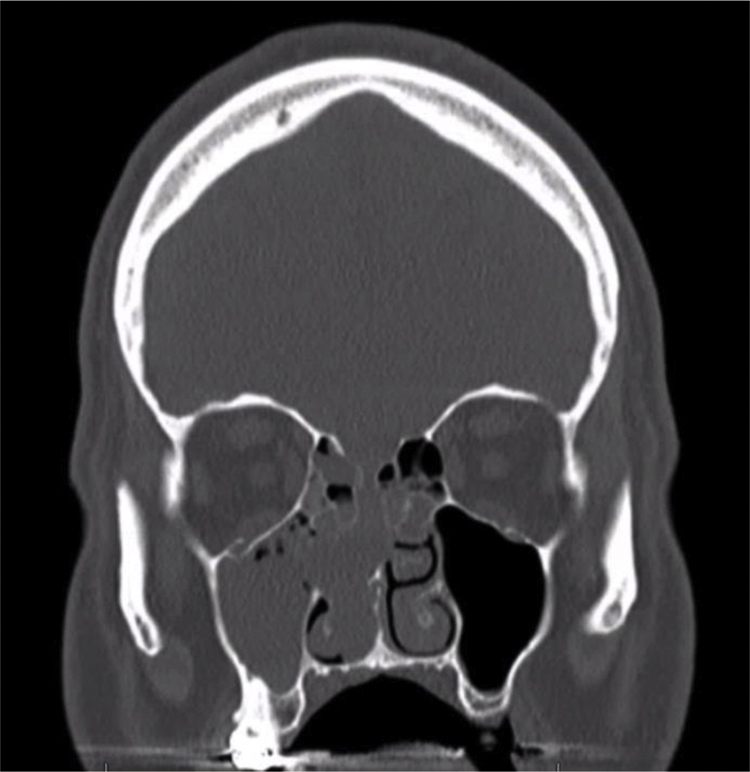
Noncontrast CT. Coronal cut demonstrating complete opacification of the right maxillary sinus with partial opacification of the ethmoid air cells as well as thinning of the skull base.
